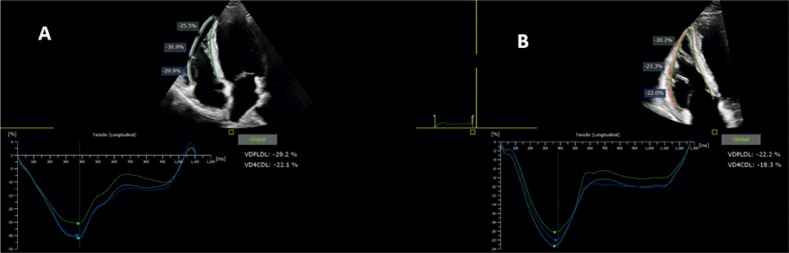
Protocolo de imagem para medir a deformação do ventrículo direito (VD) por meio da ecocardiografia speckle tracking na projeção apical 4 câmaras. A) Janela apical 4 câmaras (forma de aquisição inadequada); B) Janela apical 4 câmaras focada no VD (forma de aquisição adequada). VD4CDL: strain global longitudinal do ventrículo direito; VDPLDL: strain de parede livre do ventrículo direito.
